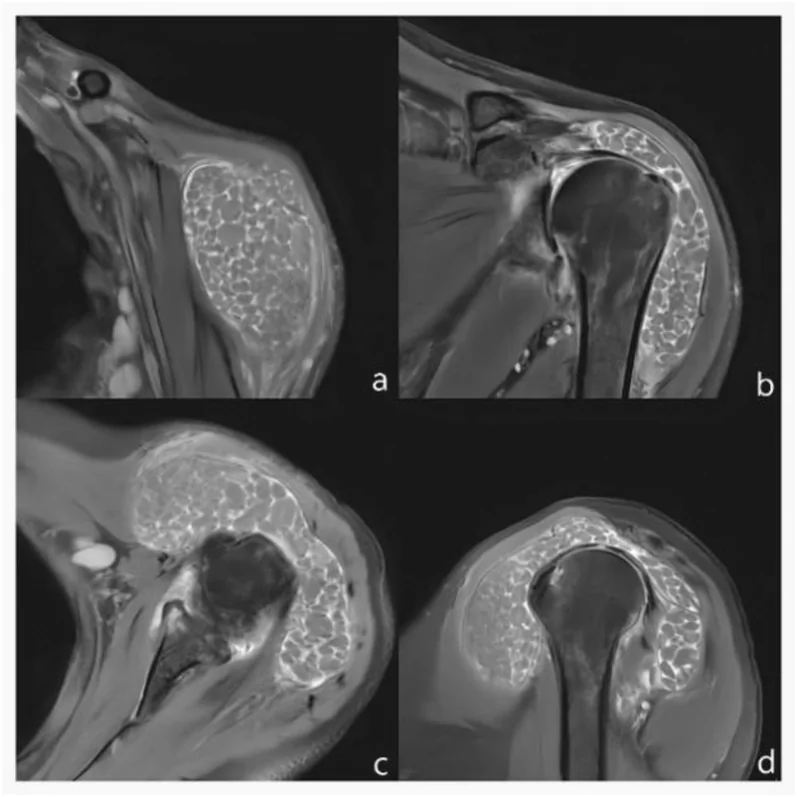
Preoperative multi-sequence MRI of the left shoulder joint in the 58-year-old male patient.
